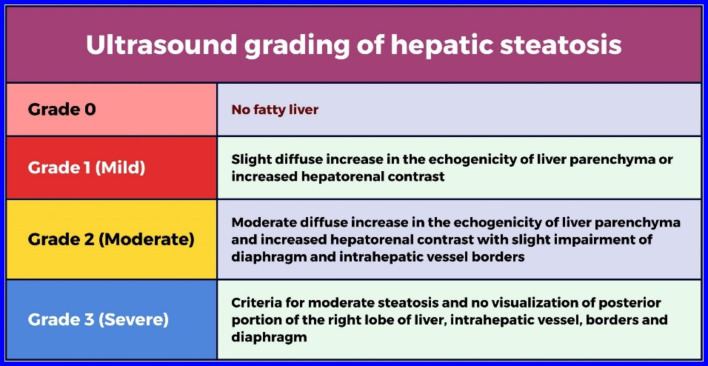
Ultrasound grading of hepatic steatosis
